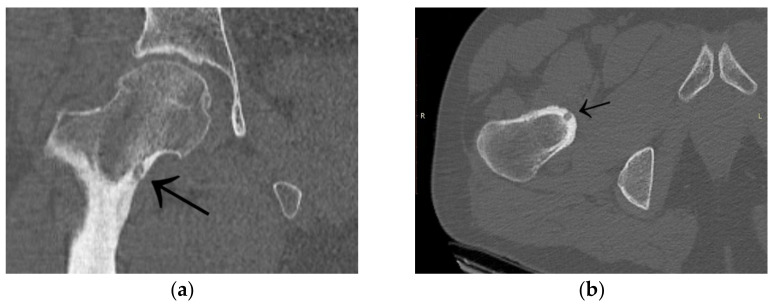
CT scan showing a focus of OO (arrows) in the right femoral neck. (a) Frontal CT scan; (b) Transverse CT scan.
